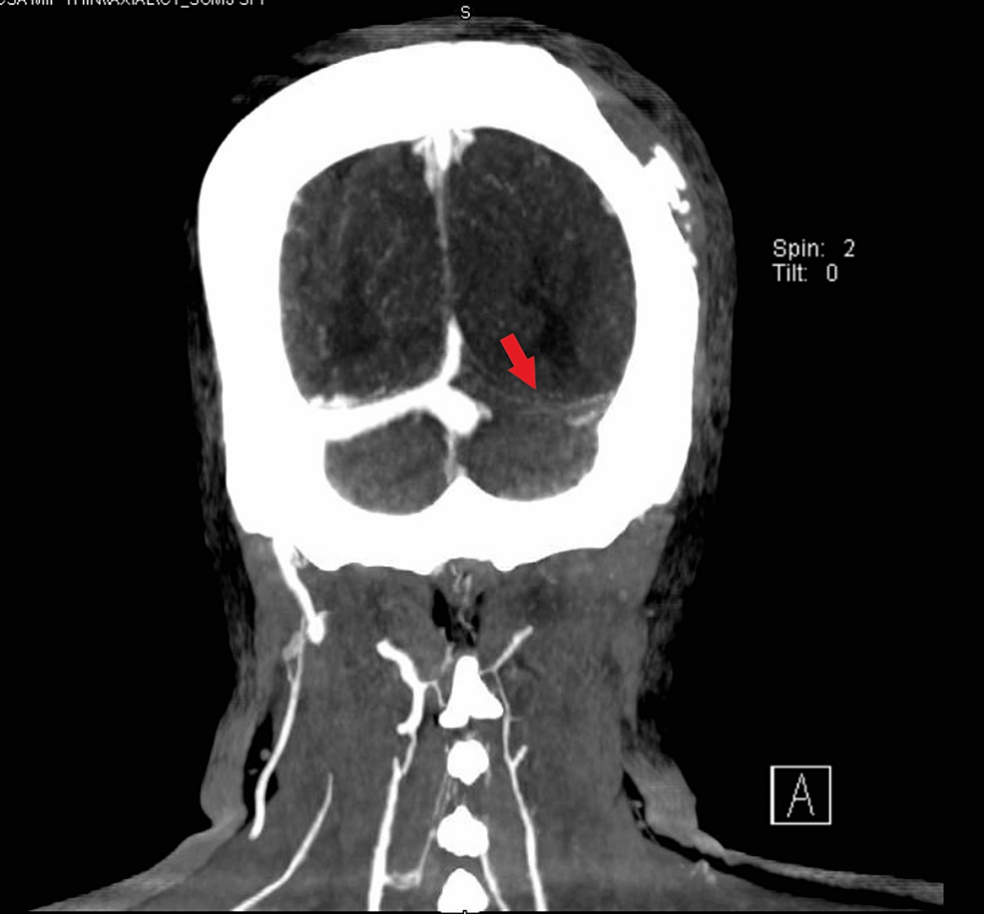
CT head venogram: arrow showing new filling defect in the left sigmoid sinus.
